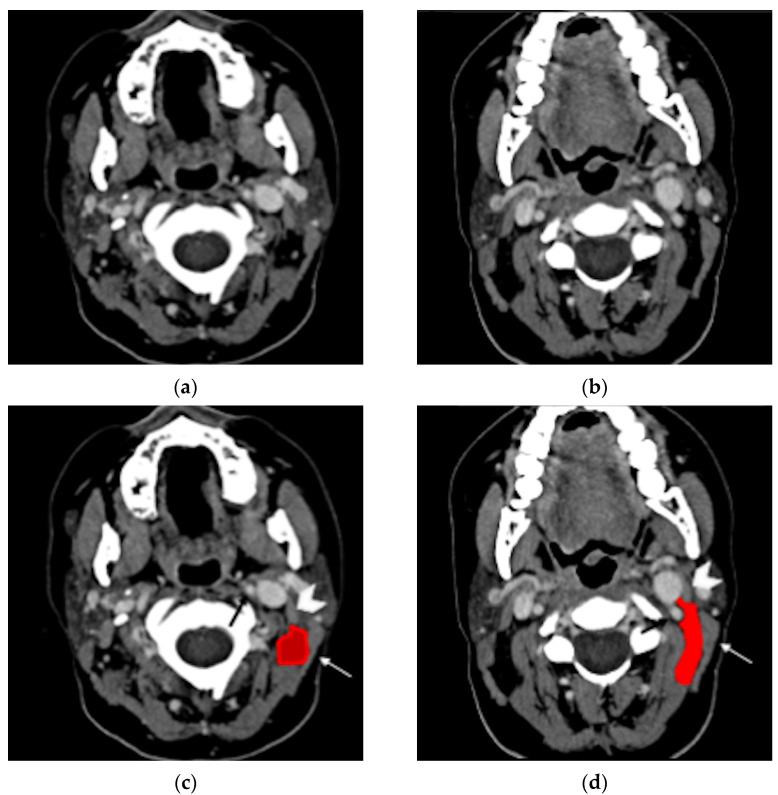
Normal anatomy, post-contrast axial CT (a,b). In the same images with annotations (c,d), the digastric muscle (white arrowhead) and the sternocleidomastoid muscle (white arrow) delimitate the posterior cervical space (red). The carotid artery can be identified nearby (black arrow).
